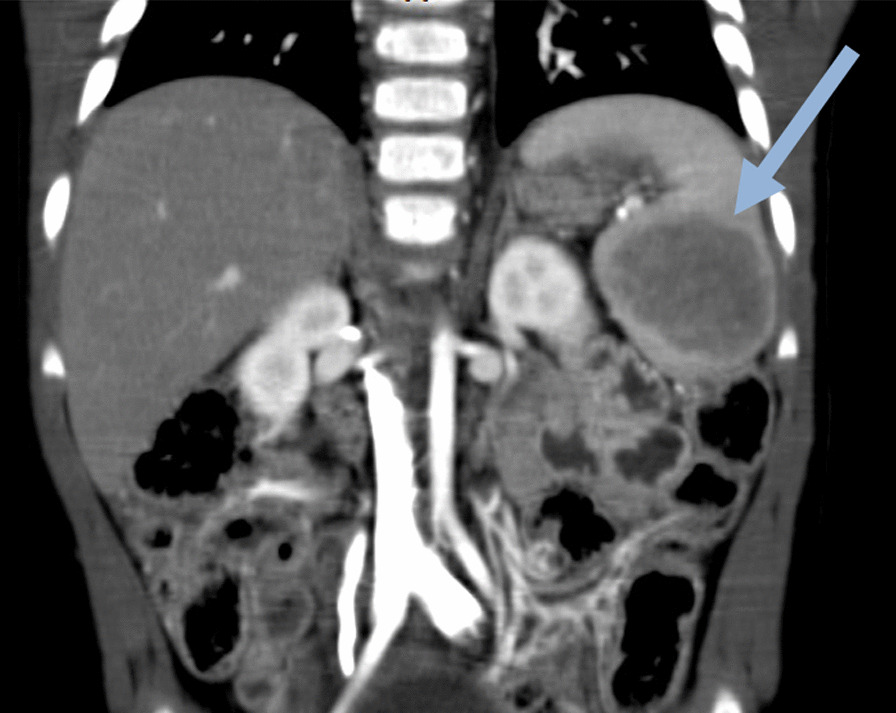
Computed tomography image of the patient shows an intrasplenic mass (arrow)
